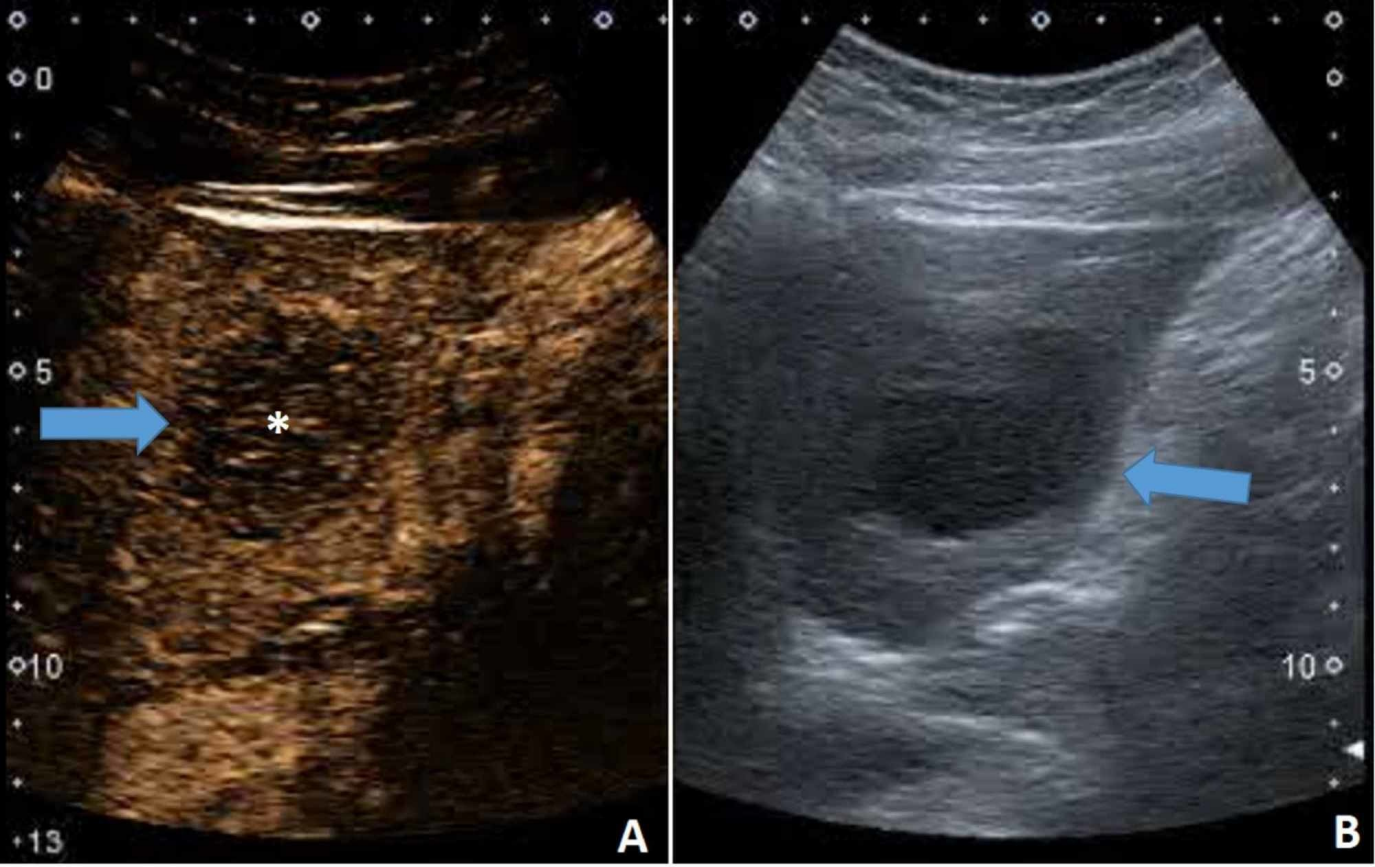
CEUS (A) demonstrating liver lesion's (arrow) microvasculature (asterisk) and fast washout. (B) B-mode US of the liver lesion (arrow). CEUS, contrast-enhanced ultrasound US, ultrasound
